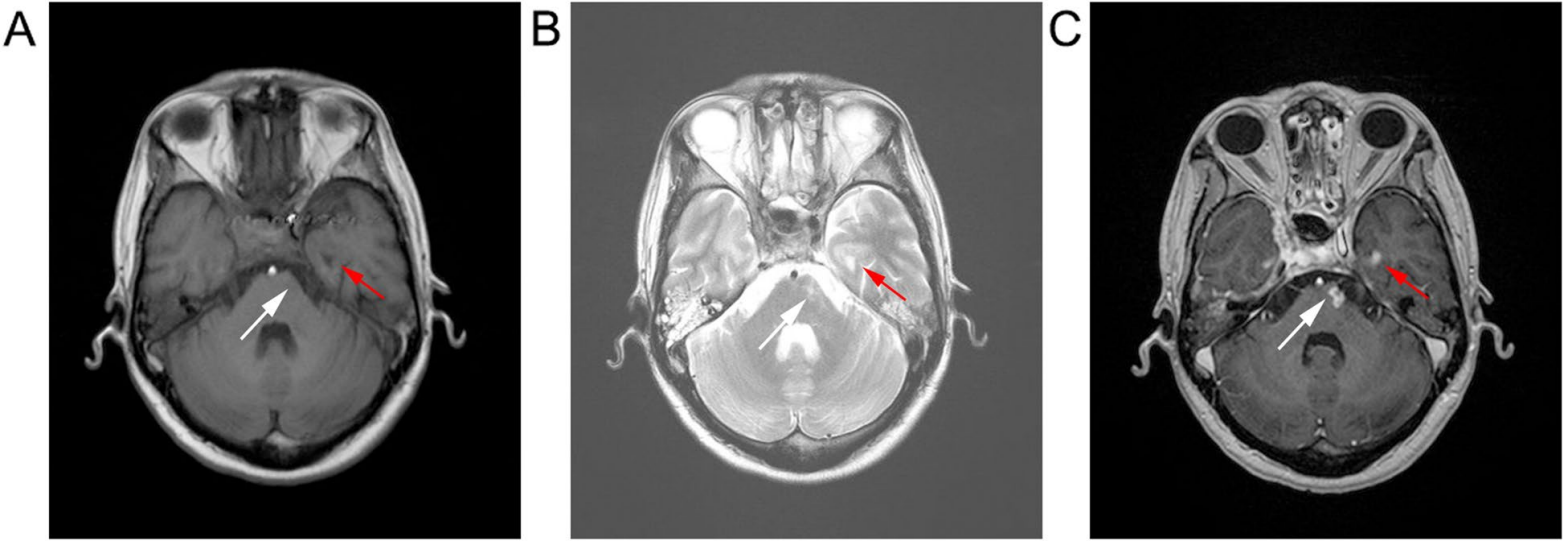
Typical presentation of brainstem necrosis on MRI of a 43-year-old man, treated with IMRT. Axial images showing marginal enhancement and surrounding edema in the pons (white arrows) and left temporal lobe (red arrows). a T1-weighted image; (b) T2-weighted image; and (c) contrast-enhanced T1-weighted image. The patient had a mild memory loss and swallowing dysfunction at 42 months of follow up
